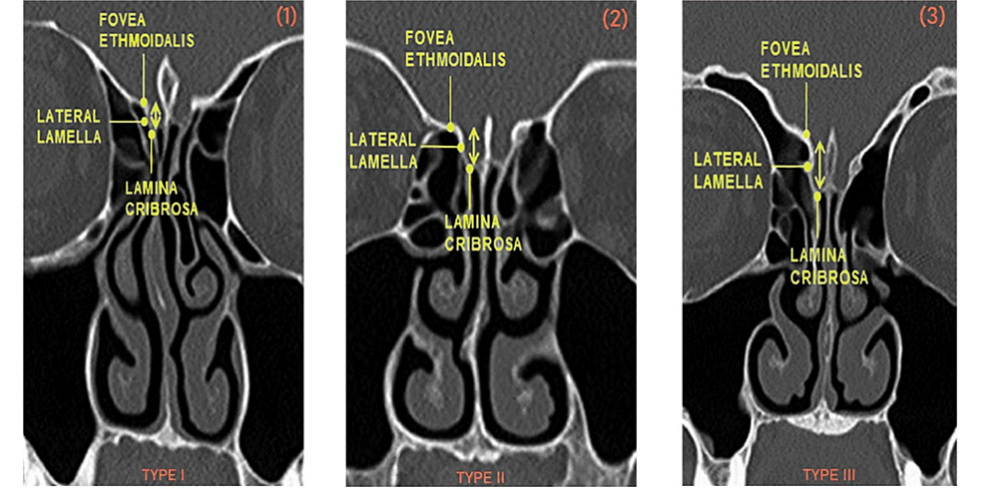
CT scans showing the Keros classification (1) Keros type I, (2) Keros type II, and (3) Keros type III
